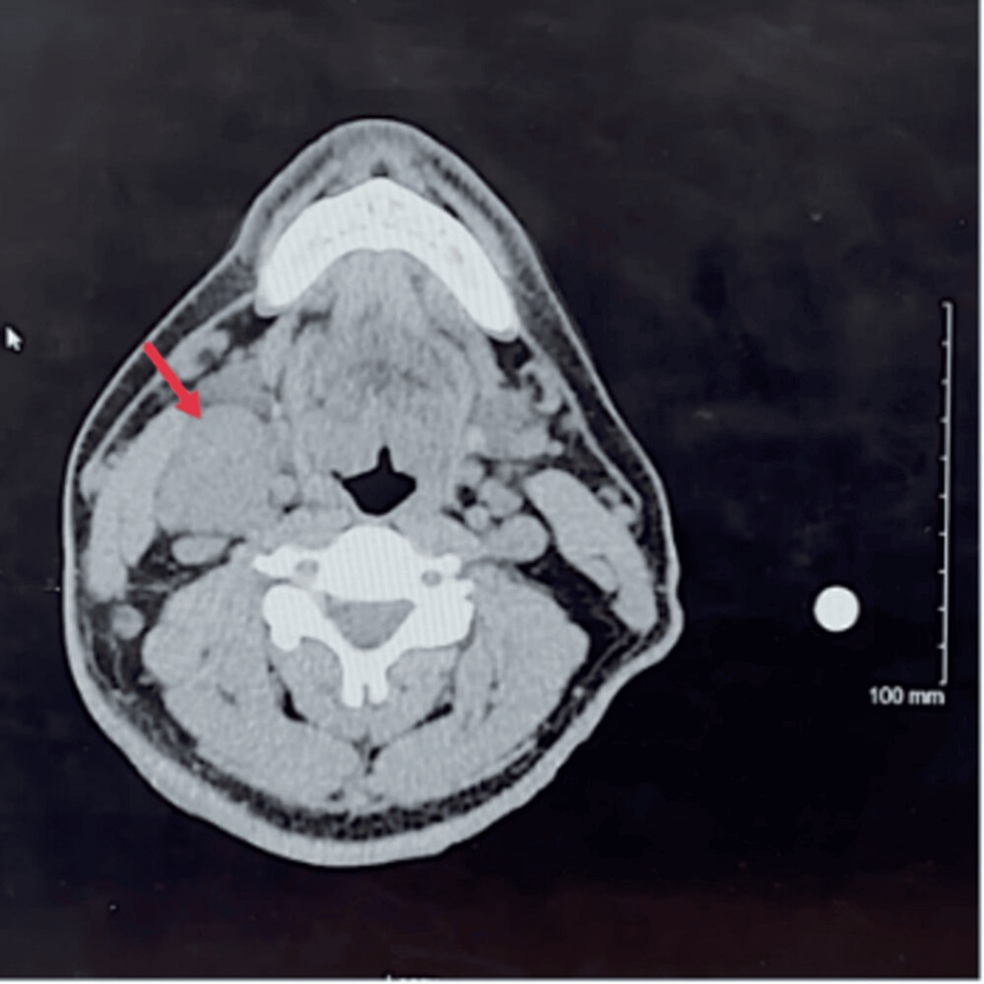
CT soft tissue neck CT soft tissue neck demonstrating a right neck soft tissue mass measuring approximately 3.8 x 2.7 x 6.0 cm.
